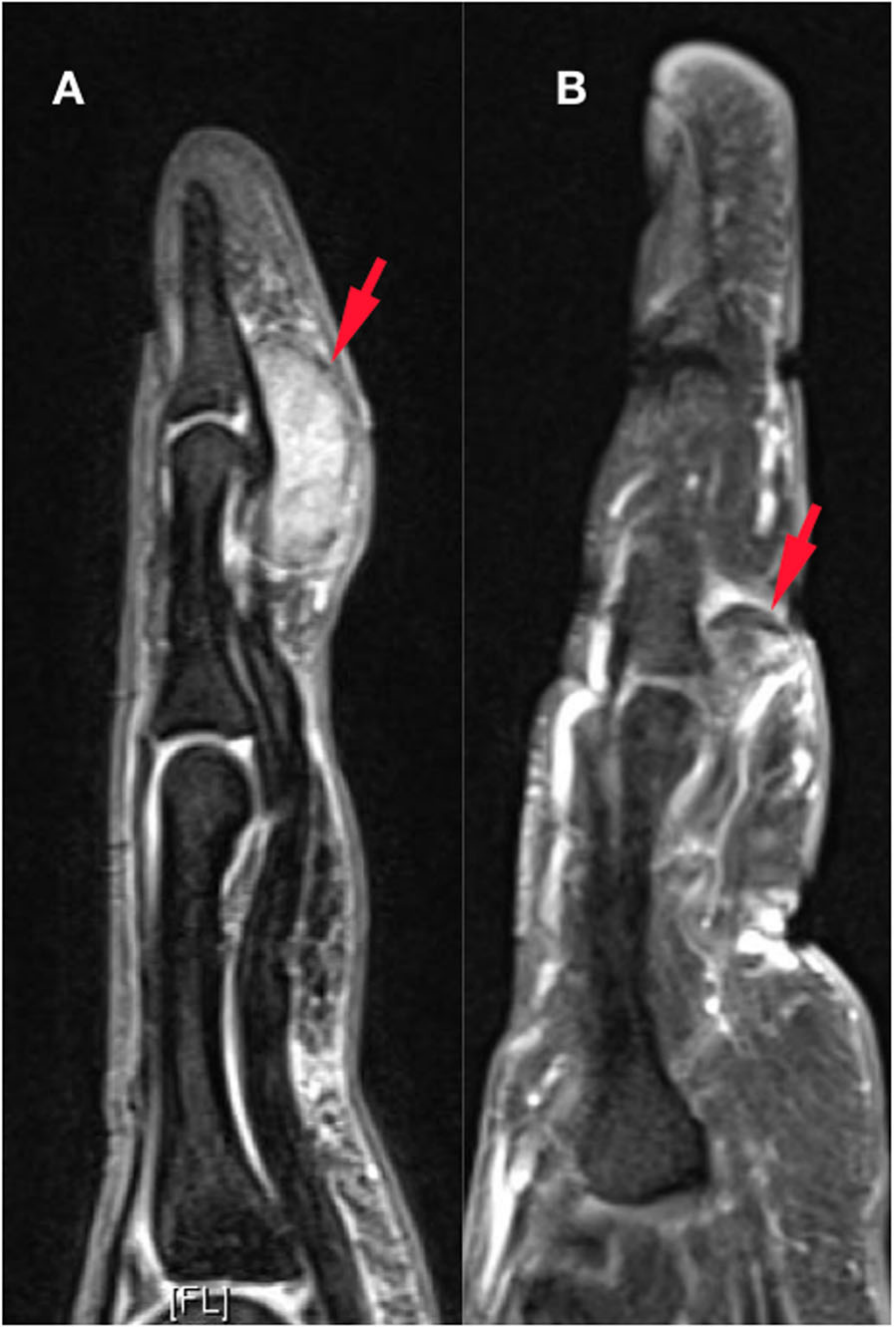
MRI may reveal GCTTS proximity to DIP (a) and PIP (b) joints
